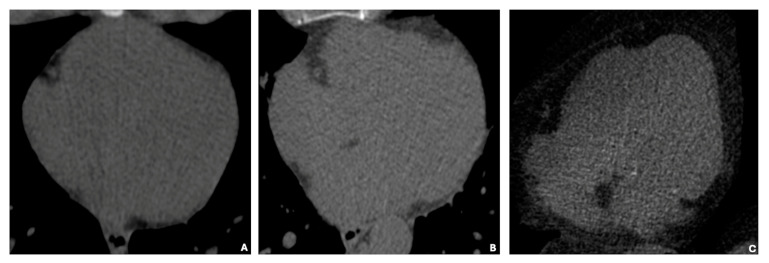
(A–C) show basal cardiac axial scans obtained through non-contrast computed tomography (NCCT) of individuals with varying epicardial adipose tissue (EAT) volumes. The images represent three distinct categories: low EAT volume (35 cm^3^) (A), average EAT volume (125 cm^3^) (B), and high EAT volume (414 cm^3^) (C).
